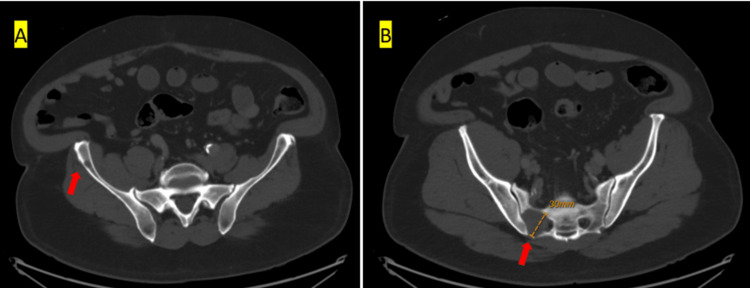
CT abdomen pelvis with contrast (A) Small lytic lesion in the right iliac wing, and 3.5 cm lytic lesion. (B) Right sacral ala without fracture
